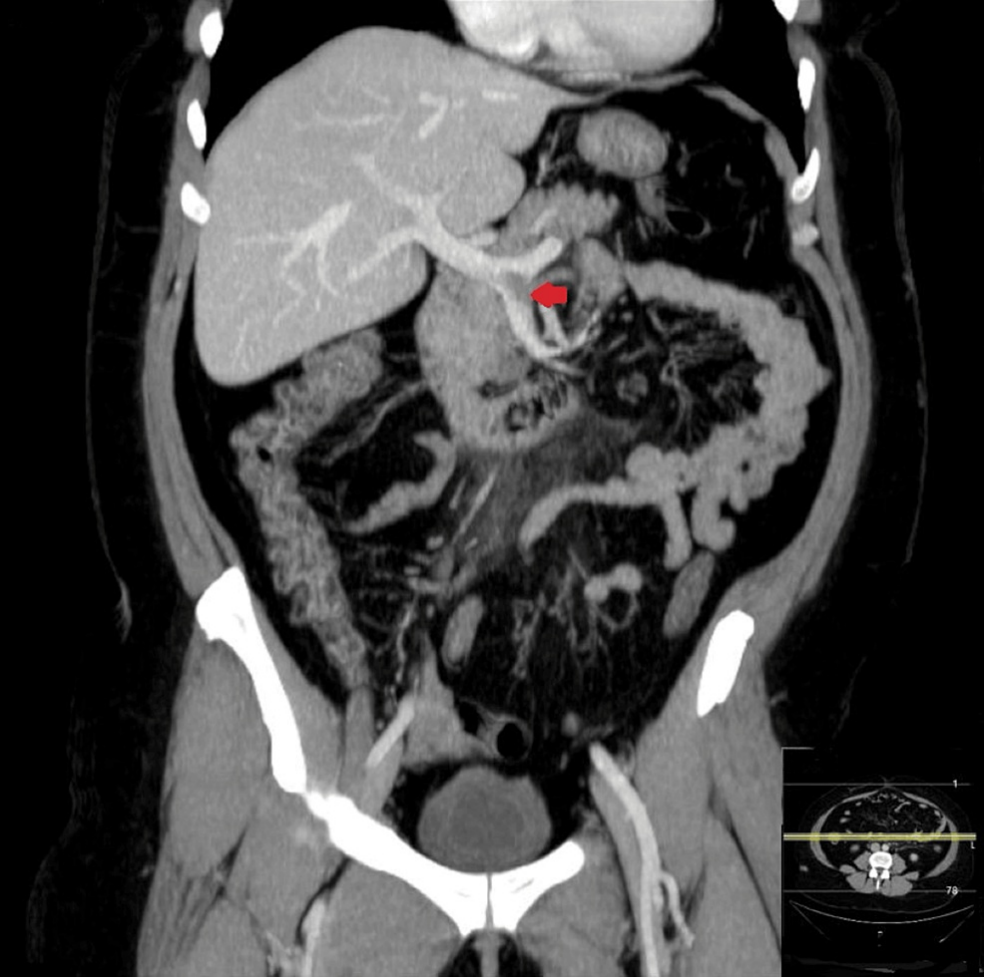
CT with coronal MIP reconstruction after the administration of intravenous iodinated contrast (portal phase). Thrombosis of the inferior mesenteric vein up to its confluence with the proximal superior mesenteric vein (anatomical variant), continuing with a partial thrombus extending to the spleno-portal confluence (red arrow). CT: computed tomography, MIP: maximum intensity projection
